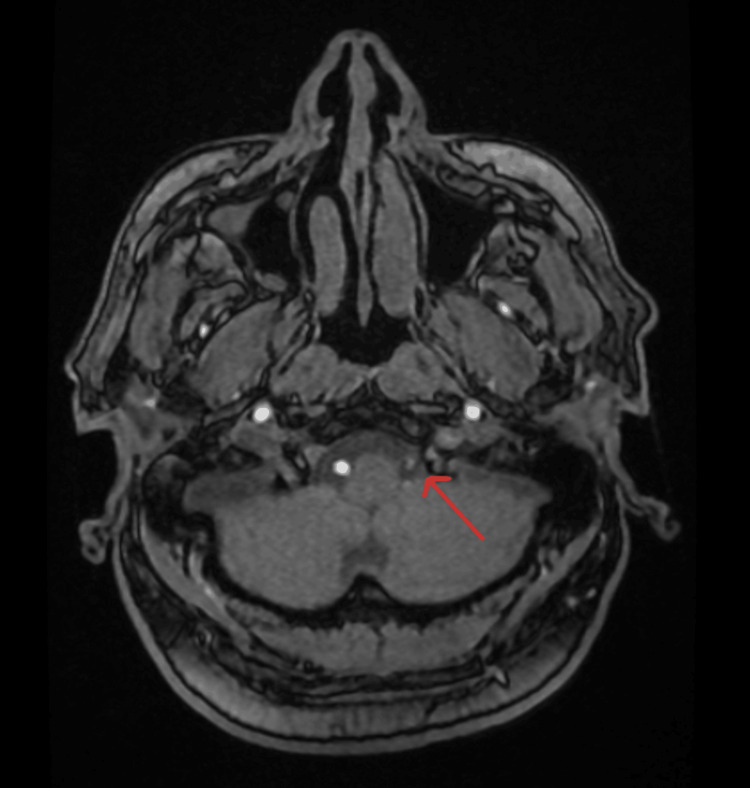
Axial T1-weighted image demonstrating measurement of the vertebral artery at the V4 segment (red arrow). The vessel caliber was recorded bilaterally on MR angiography for evaluation of vertebral artery hypoplasia and its relationship to vertebrobasilar insufficiency.
